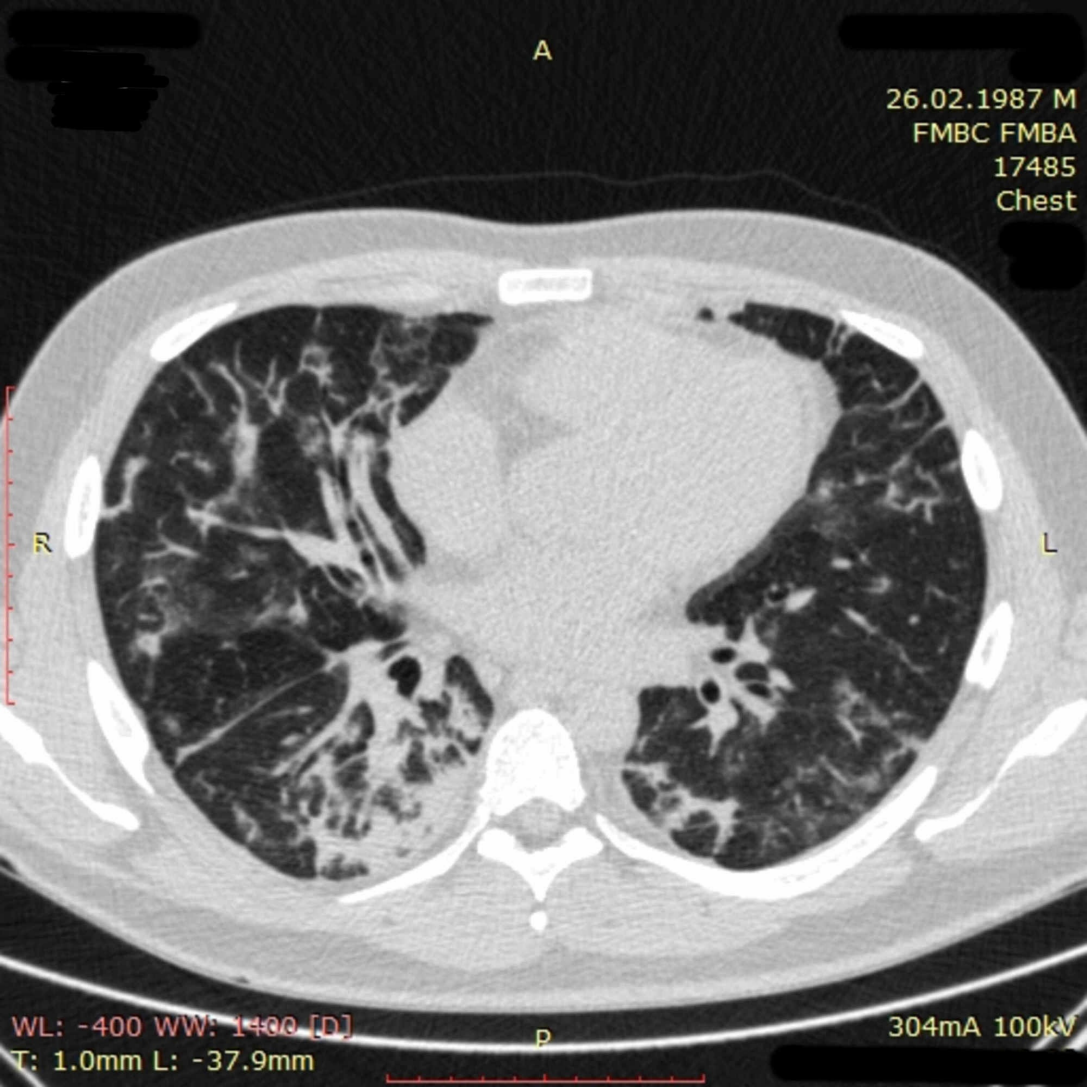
Chest CT imaging of patient 3 on day 23 Chest CT on day 23 revealed partial resolution of pulmonary tissue consolidation in the right lower lobe and a further decrease of size and density of ground-glass opacities all over the lungs CT: computed tomography
